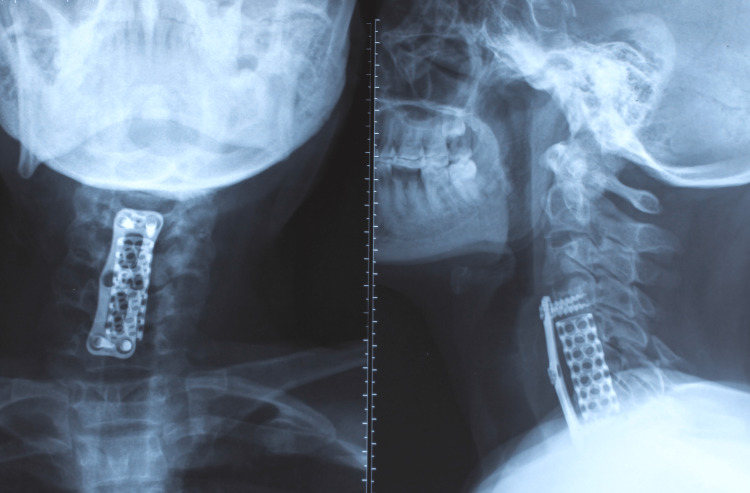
X-ray cervical spine AP & lateral view showing operative fixation
